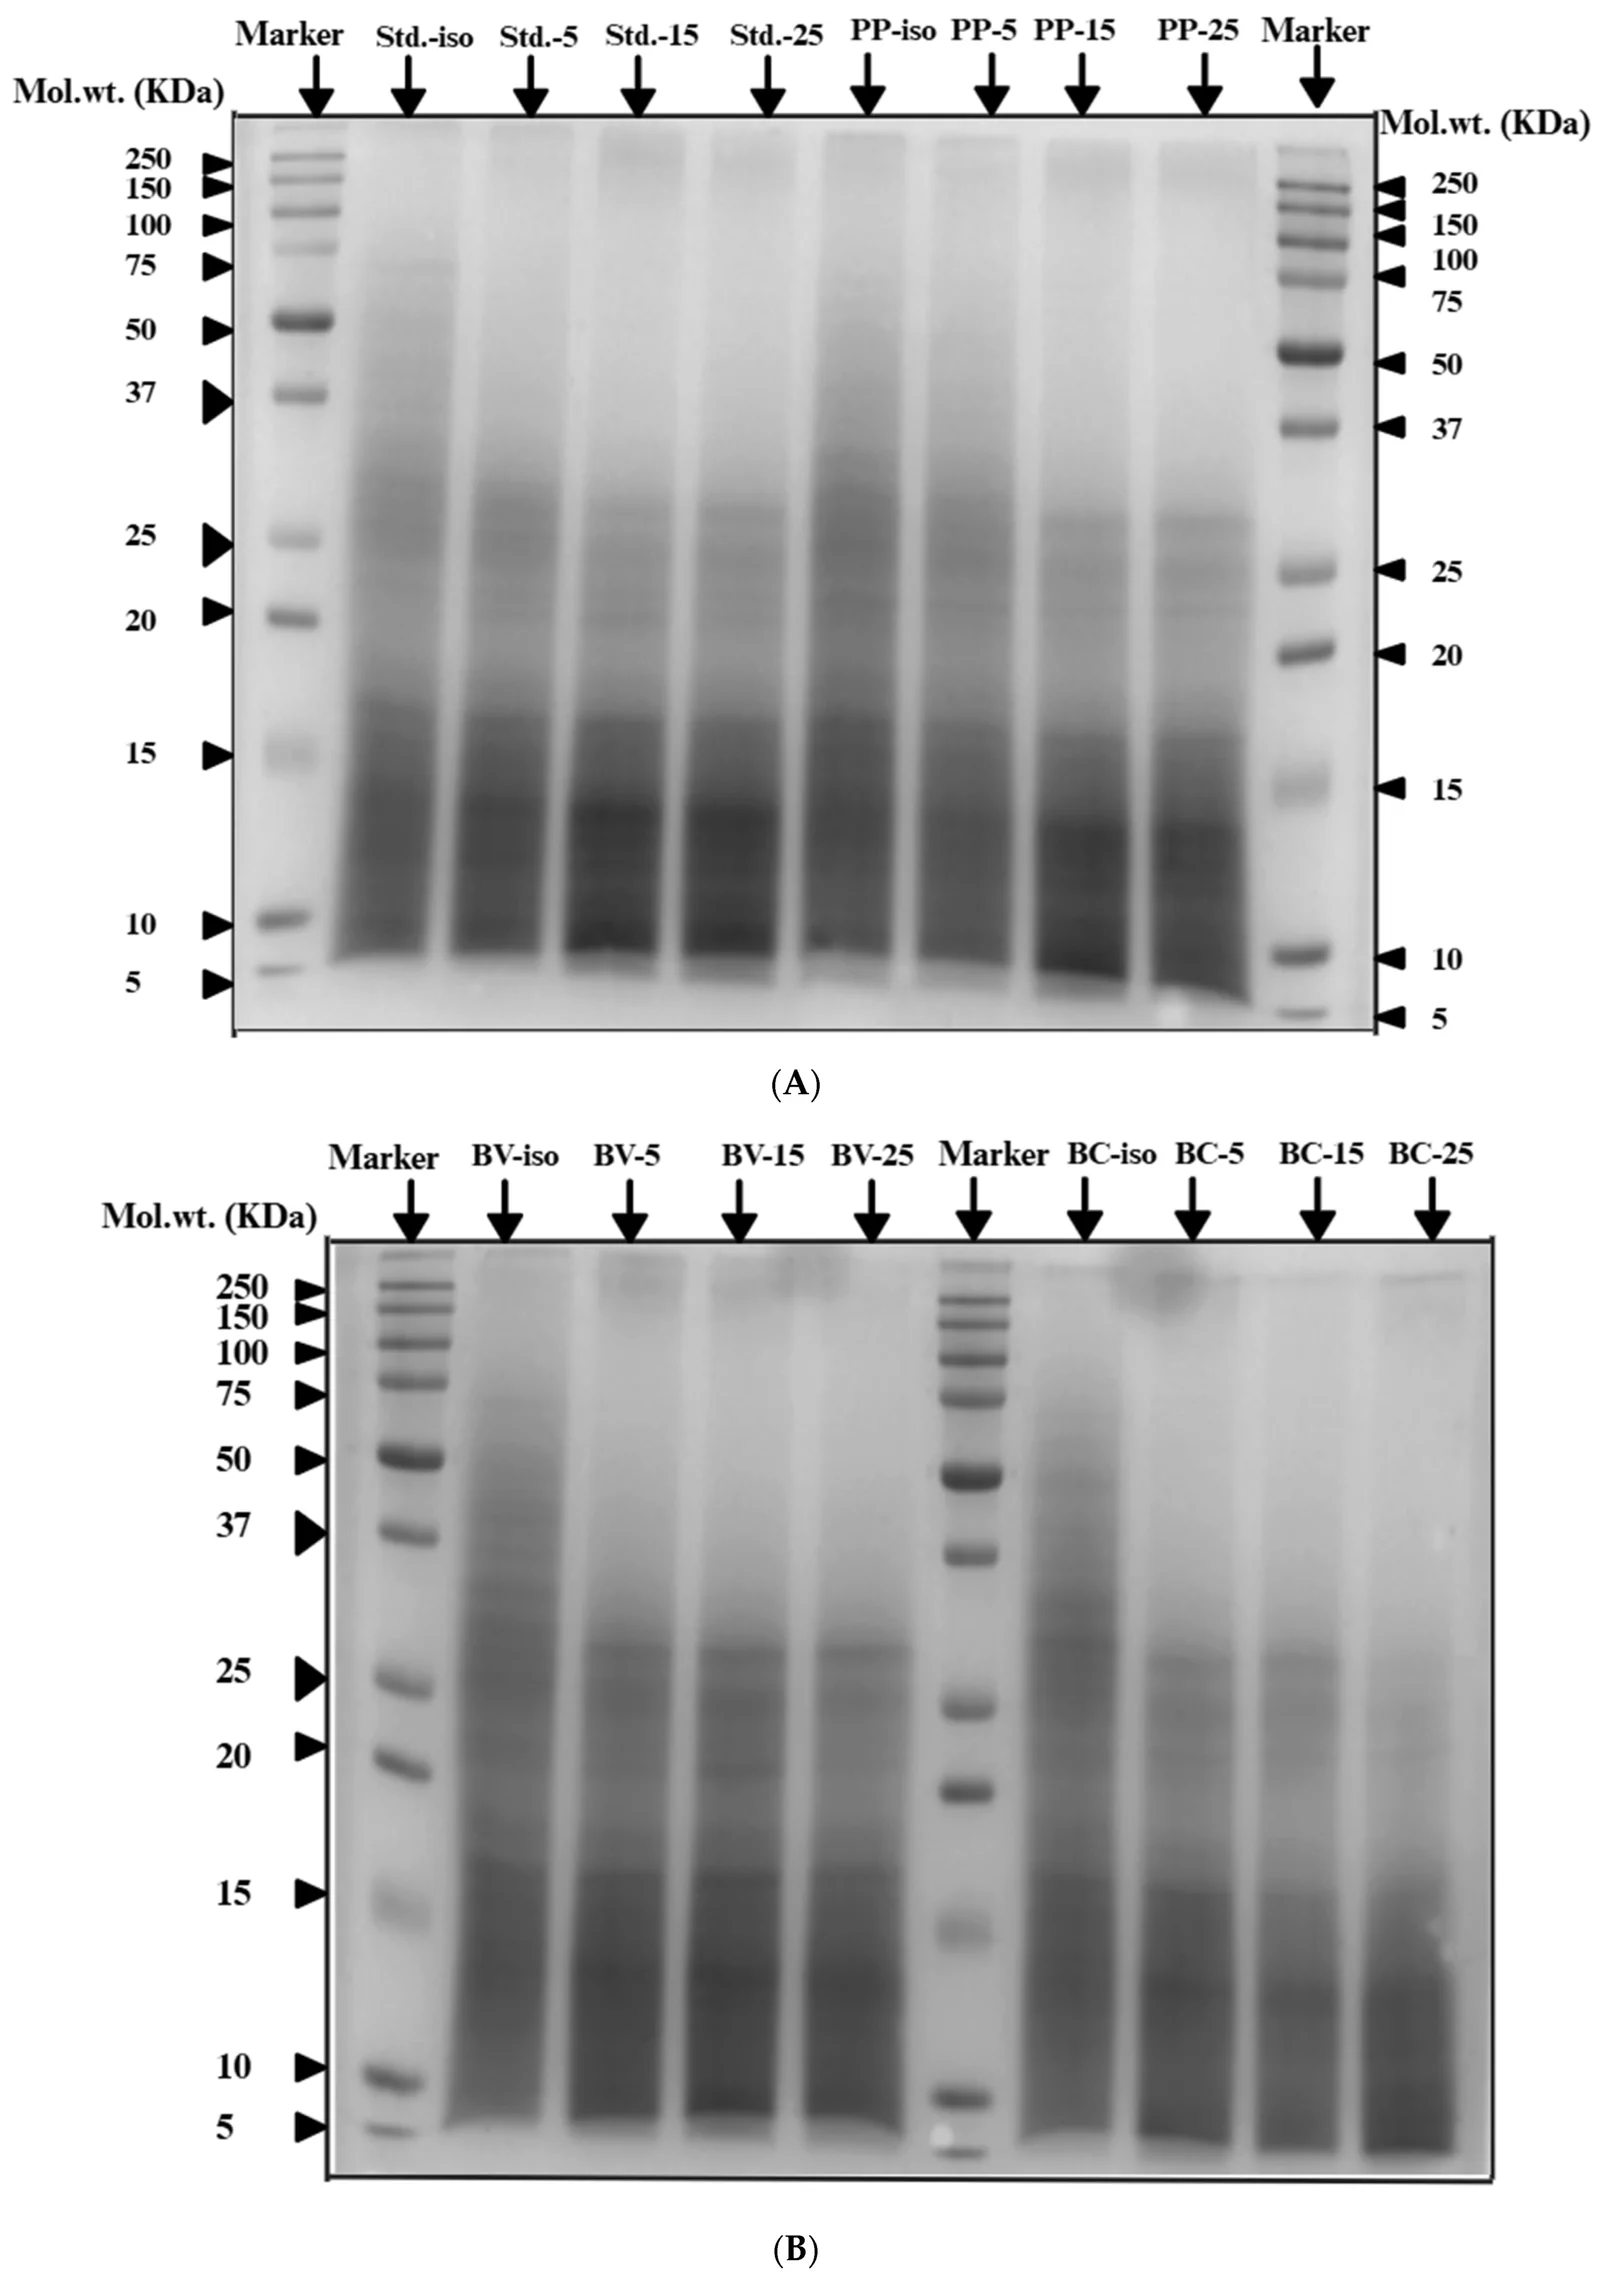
SDS-PAGE profiles of proteins extracted from yellow mealworm larvae subjected to different dietary and ultrasound treatment conditions. (A) Standard diet and *Pediococcus pentosaceus*-supplemented diet: Standard-Iso (control), Standard-5, Standard-15, Standard-25, PP-Iso (control), PP-5, PP-15, and PP-25. (B) *Bacillus velezensis*- and *Bacillus coagulans*-supplemented diets: BV-Iso (control), BV-5, BV-15, BV-25, BC-Iso (control), BC-5, BC-15, and BC-25. UST, ultrasound treatment; Iso, isoelectric precipitation control.
